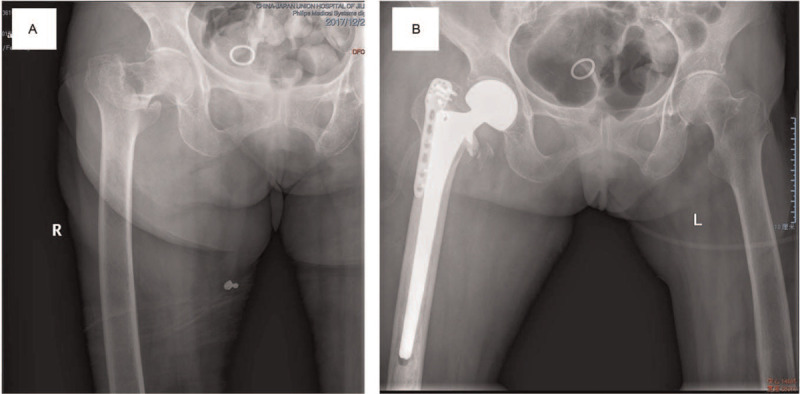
An 84-year-old woman had right hip pain after fall down injury. (A) Pelvis anteroposterior radiograph shows intertrochanteric fracture with obvious osteoporosis. (B) Postoperative radiographic shows she received a HA and the greater trochanter fixed with proximal humeral locking plate. HA = hemiarthroplasty.
